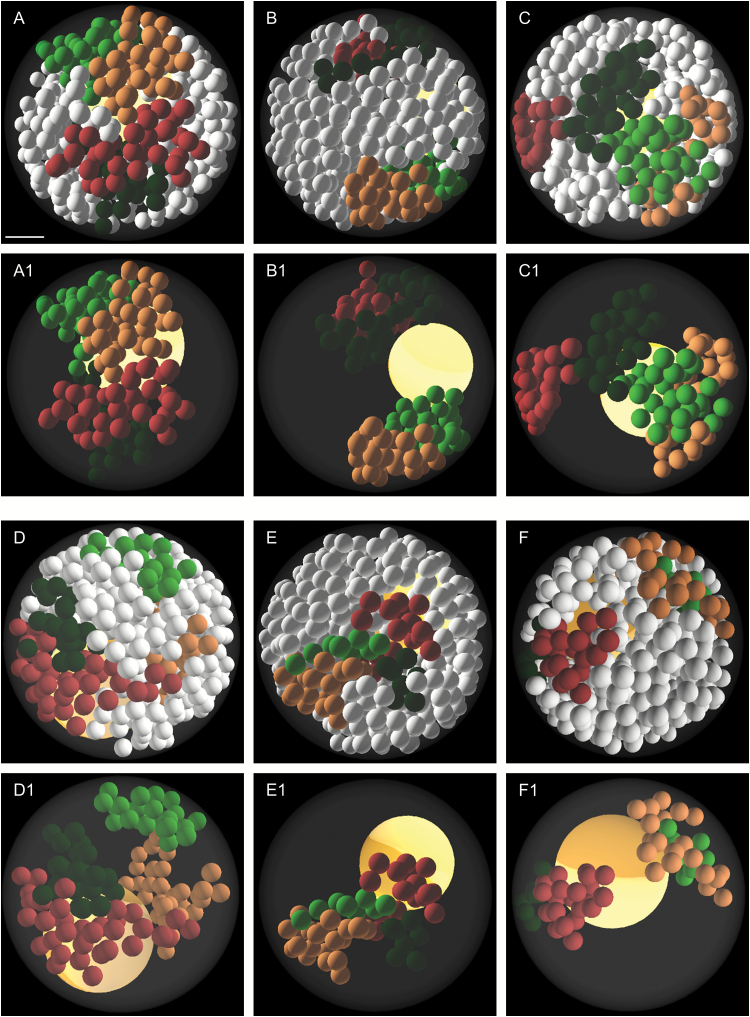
Exemplary visualization of nuclei modelled using ChroTeMo. (A, B) Nuclei with a complete association (A) or separation (B) of Bd1 chromosome arm territories. (C) Nucleus with a Bd2 top arm CT association. (D) Nucleus with a Bd3 bottom arm CT association. (E, F) Nuclei with a complete association (E) or separation (F) of Bd5 chromosome arm territories. In images (A–F), chromosomes other than the analysed pair are coloured in white. In images (A1–F1), chromosomes other than the analysed pair are transparent. One of the homologues in each image is coloured with darker shades of green and red, and the other one with lighter shades.The nucleolus is visible as a yellow sphere inside the nucleus. Scale bar=1 µm.
